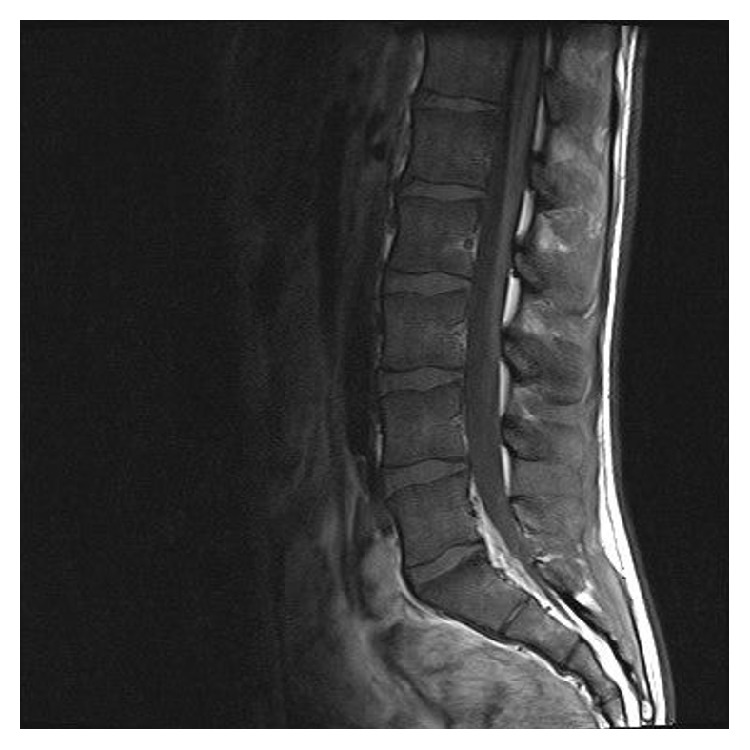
MRI of the spine showing lesions in lumbar spine body 2.
